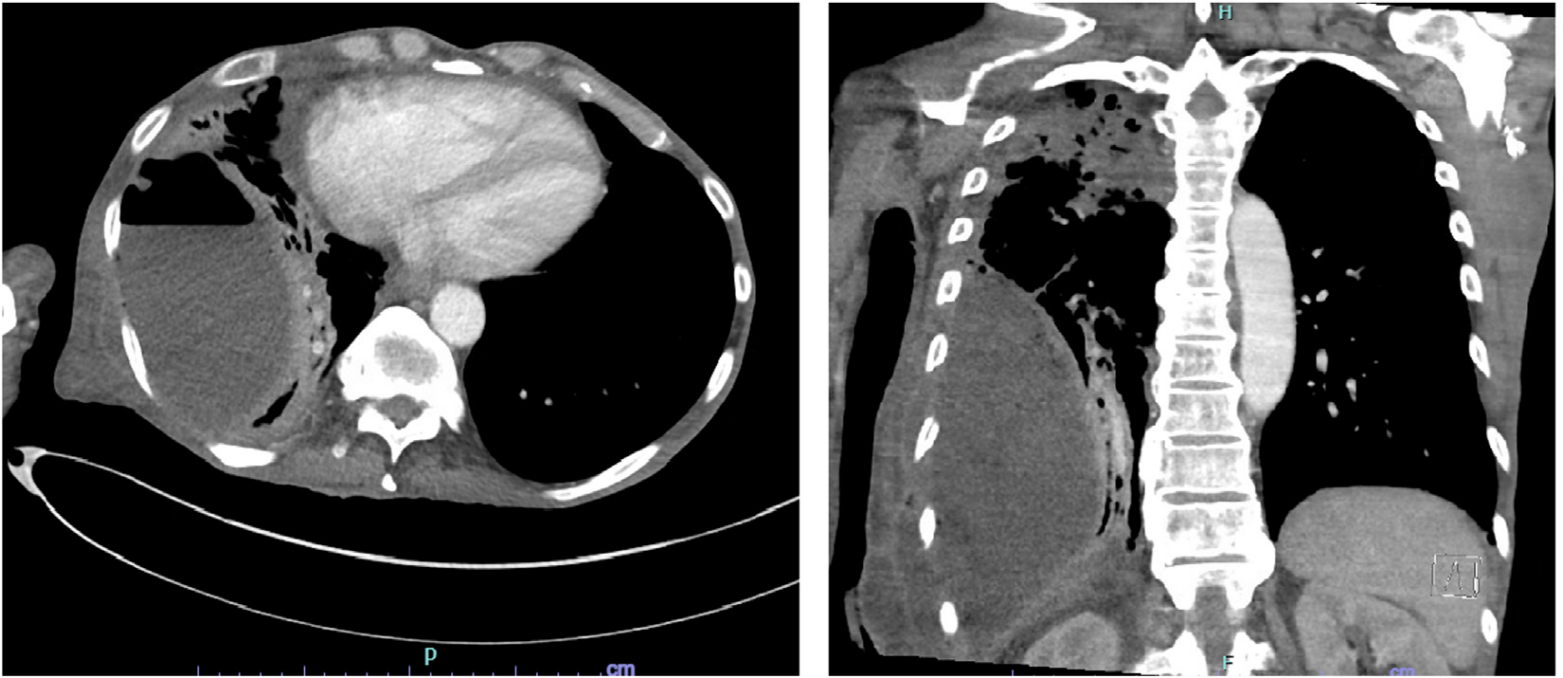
Computed tomography imaging demonstrated a 1-L collection lateral to the right lower lobe containing an air-fluid level. Multifocal consolidation and scarring to the right lung were noted.
